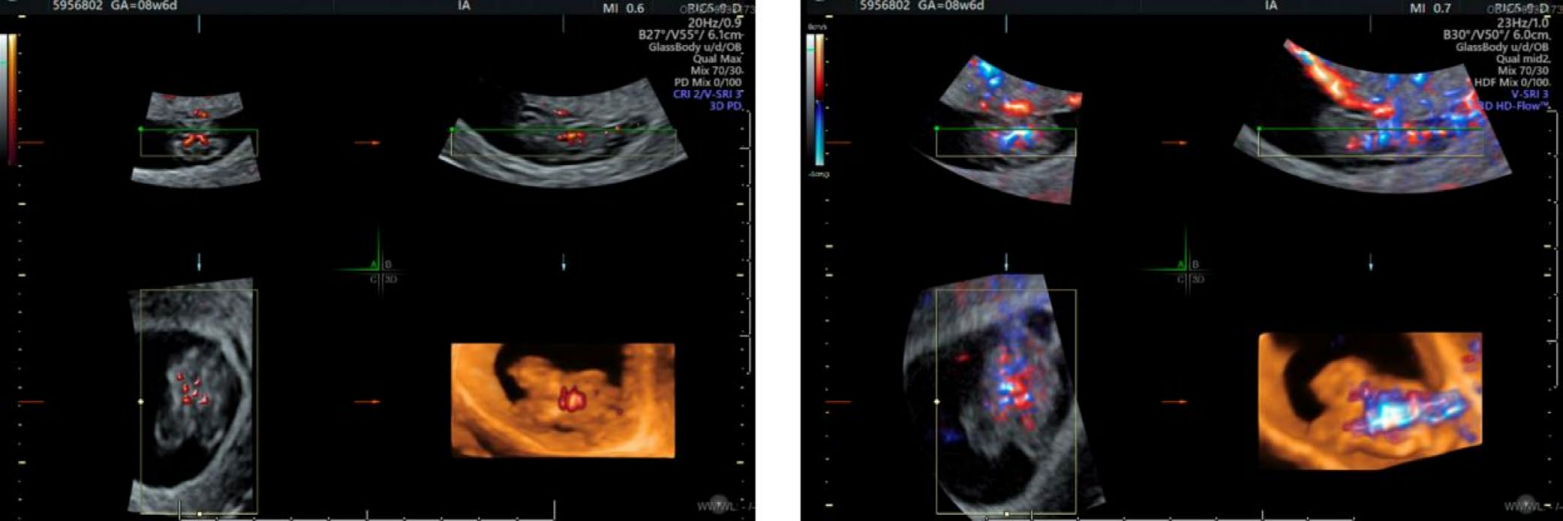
3D ultrasonography of thoraco‐omphalopagus conjoined twins at 8 weeks and 6 days.
